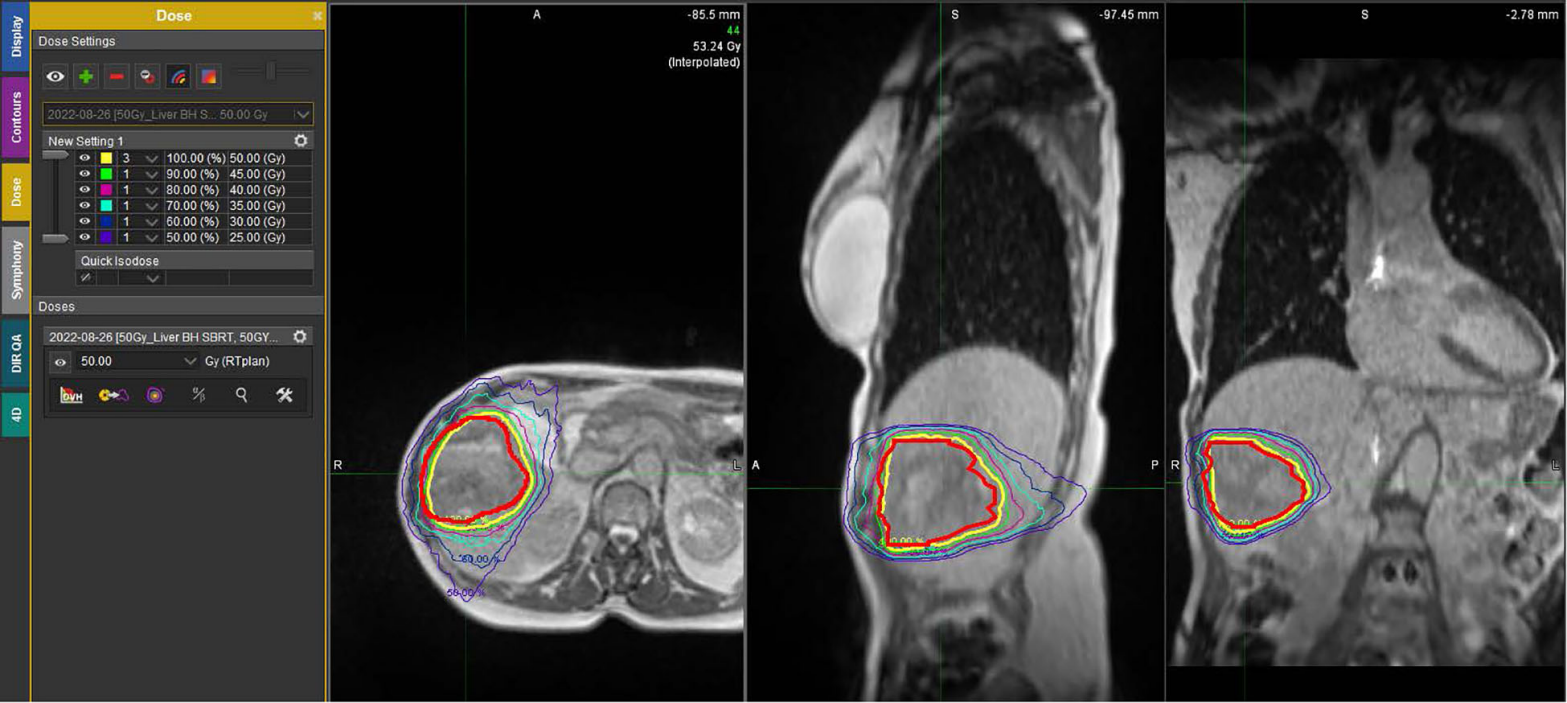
Example of the dose distribution on MRI from the SBRT course, composed of five 10-Gy fractions delivered over consecutive days. The transverse, sagittal, and coronal views demonstrate the isodose levels from 50 Gy (prescription) to 25 Gy.
